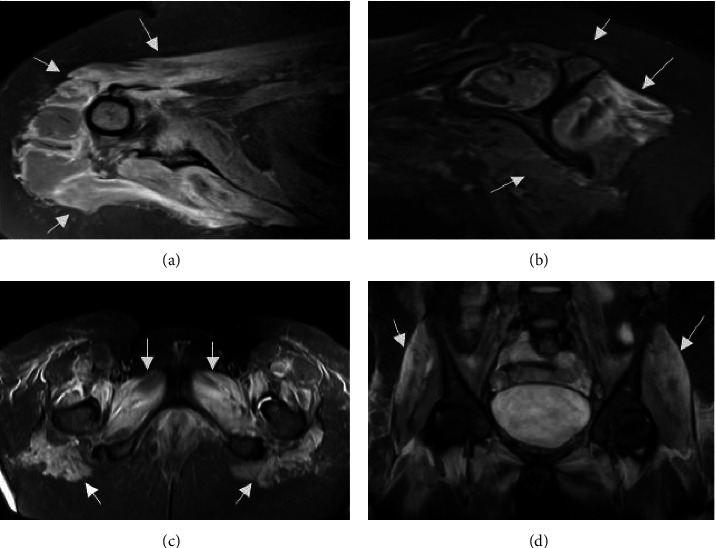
(a) Magnetic resonance imaging (MRI) of the shoulder, oblique axial view, DP sequence, with fat saturation, showing hyperintensity in the m. pectoralis major and m. deltoid. (b) MRI of the shoulder, oblique sagittal view, T2 sequence with fat saturation, showing hyperintensity in the rotator cuff muscles (supraspinatus, infraspinatus, and subscapularis). (c) MRI of the pelvis, axial section, and STIR sequence, showing hypersignal in the adductor and extensor muscles of the thigh and in the gluteus maximus. (d) MRI of the pelvis, coronal section, and STIR sequence, showing hyperintensity in the thigh extensor musculature.
